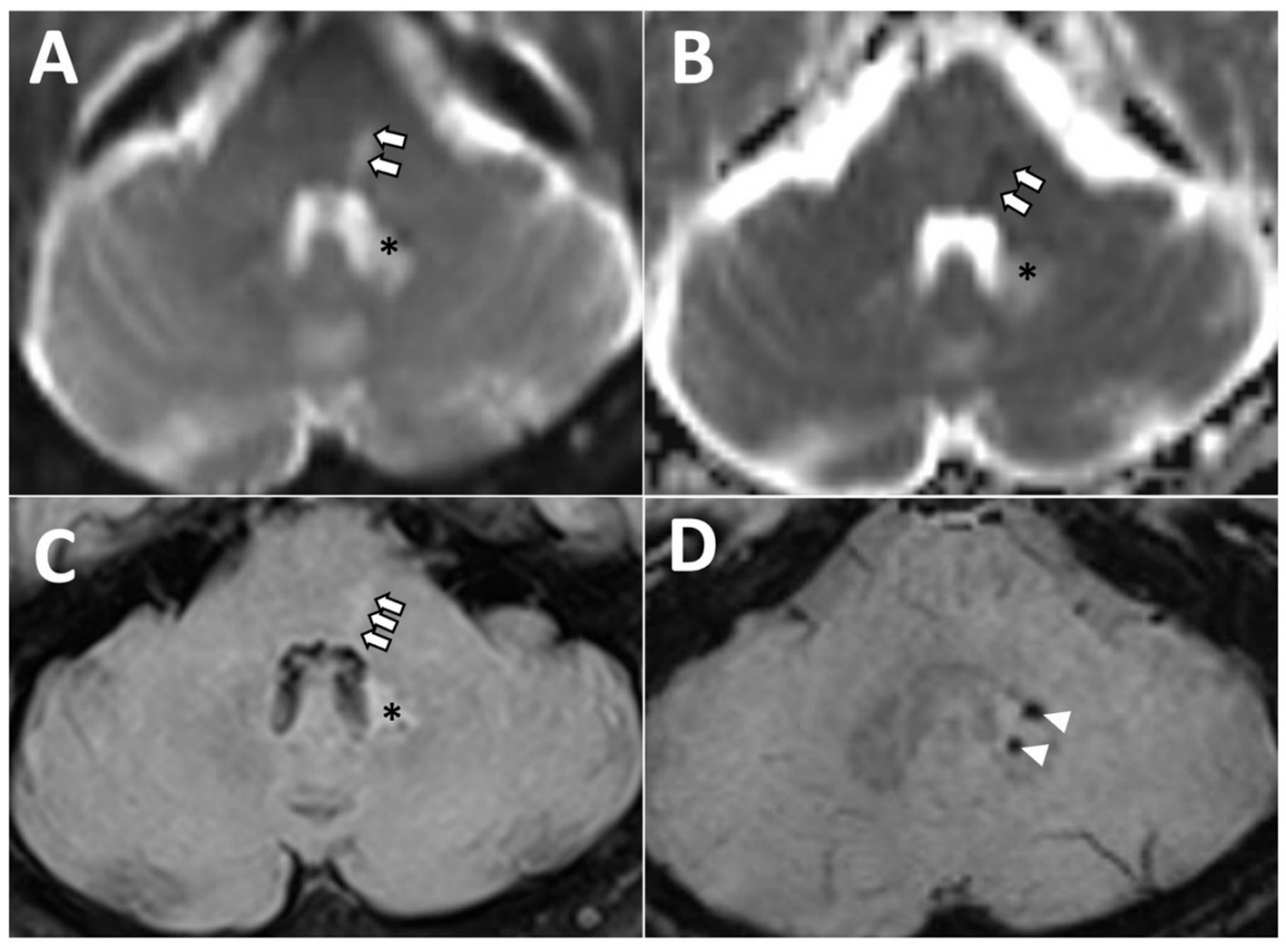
Axial magnetic resonance images of the posterior fossa demonstrating left dorsal hemi-pontine signal change (arrows) on diffusion-weighted (A), apparent diffusion coefficient (B), and fluid-attenuated inversion recovery (FLAIR) (C), consistent with acute ischaemia in the territory of a perforating artery, and likely involving left lateral lemniscus and abducens nucleus. *Hyperintensity area associated with two microbleeds, as visualized on FLAIR (C) and susceptibility-weighted [arrowheads, (D)] images reflecting previous hemorrhage and gliotic changes.
